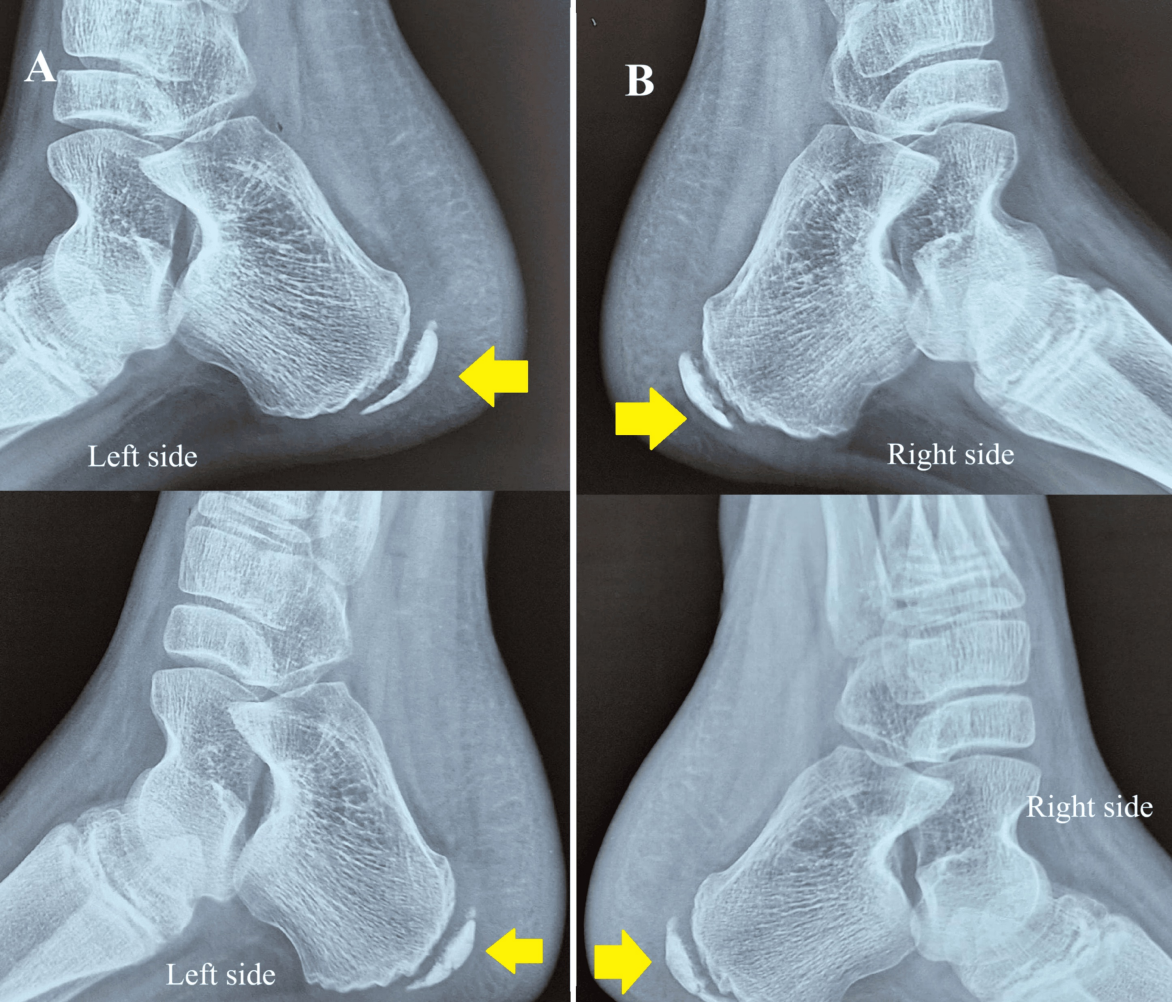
X-rays of the right and left calcaneus in both twins (lateral view). A) Lateral view X-ray of the left calcaneus in Twin 1 (top image) and Twin 2 (bottom image), showing increased density and fragmentation of the calcaneal apophysis (yellow arrow);
